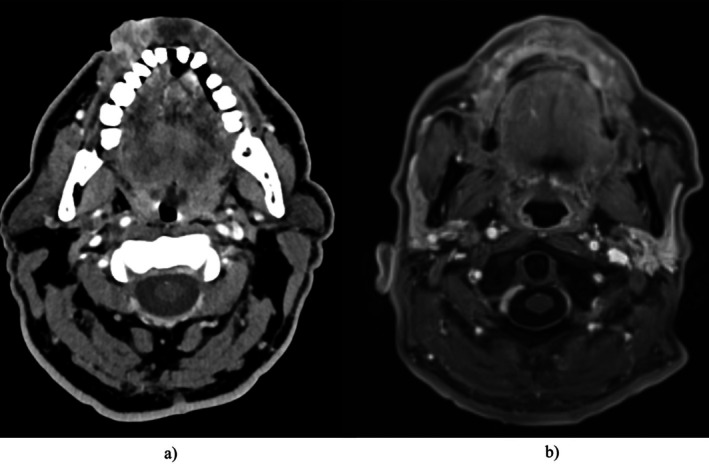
Evaluation using pre‐treatment CT (a) and post‐treatment MRI (b).
